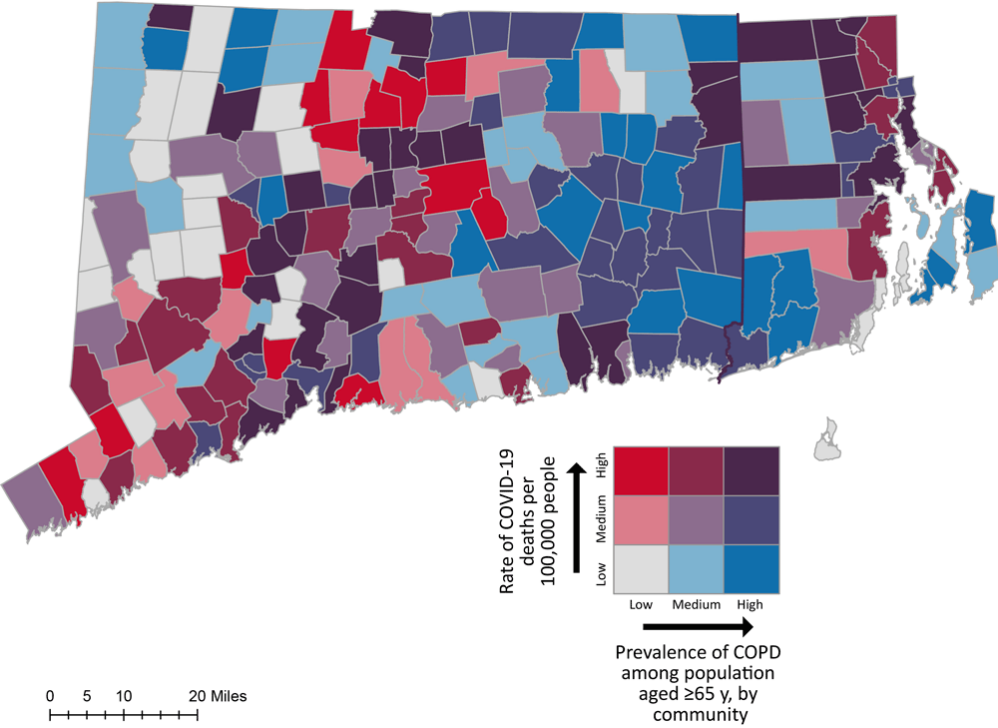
Town-level prevalence of COPD among population aged 65 years or older and COVID-19 death rates per 100,000 people, Connecticut and Rhode Island. Data sources: Connecticut Department of Public Health (8), Rhode Island Department of Public Health (9), HealthyAgingDataReports.org (10,11), CT DEEP GIS (22), and RIGIS (23).
